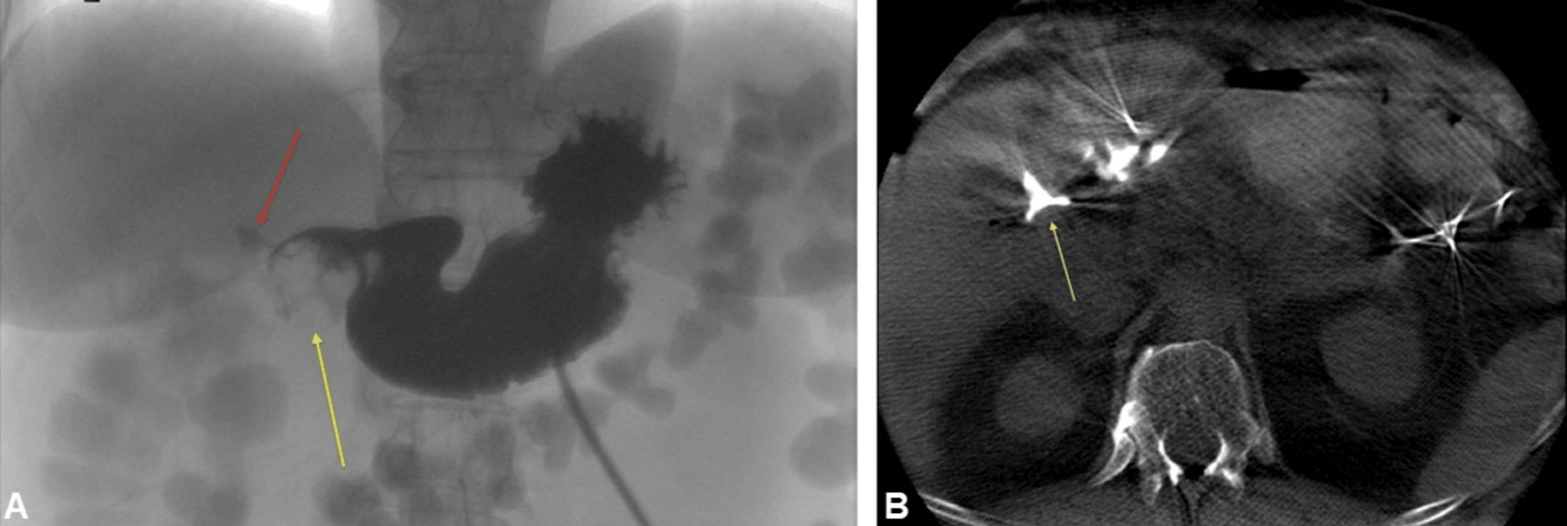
(A) Upper GI study showing a radiolucent round structure (yellow arrow) in the pylorus/first portion of the duodenum. The red arrow points to contrast extension to the gallbladder fossa in the context of a cholecystogastric/duodenal fistula. (B) Initial cone beam CT enterography with contrast injection through the gastrostomy tube, demonstrating contrast extension to the contracted gallbladder, indicating fistulous communication (yellow arrow).
